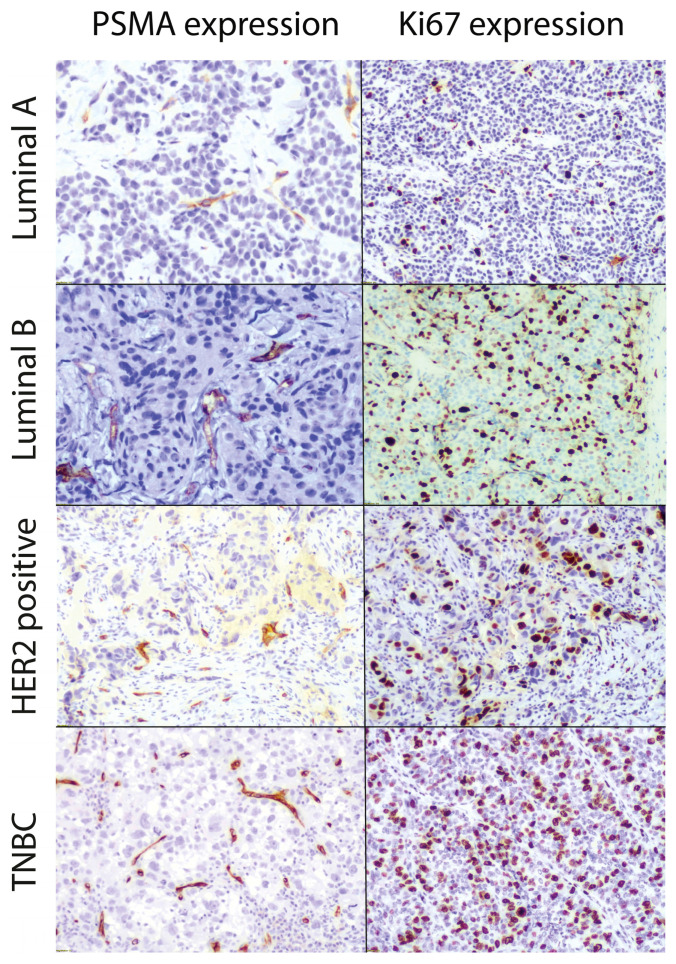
PSMA and Ki67 IHC analysis. PSMA and Ki67 expression in the different breast cancer subtypes (10x magnification).
